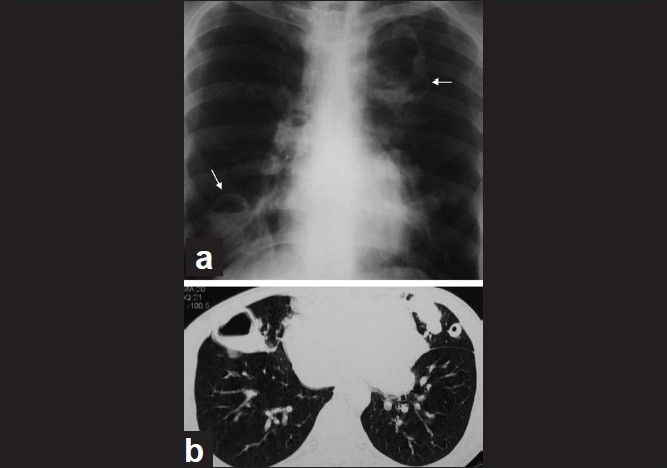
(a) Chest X-ray showing lung opacity and cavities (arrows); (b) CT scan of lungs showing cavities and nodules
